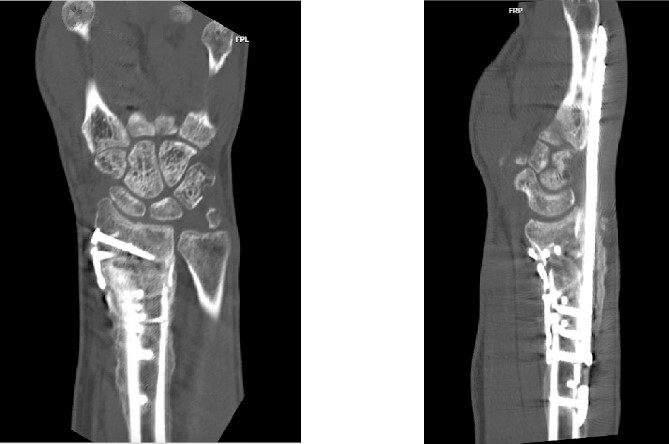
CT assessment at 10 weeks postprocedure with coronal and sagittal cuts.
